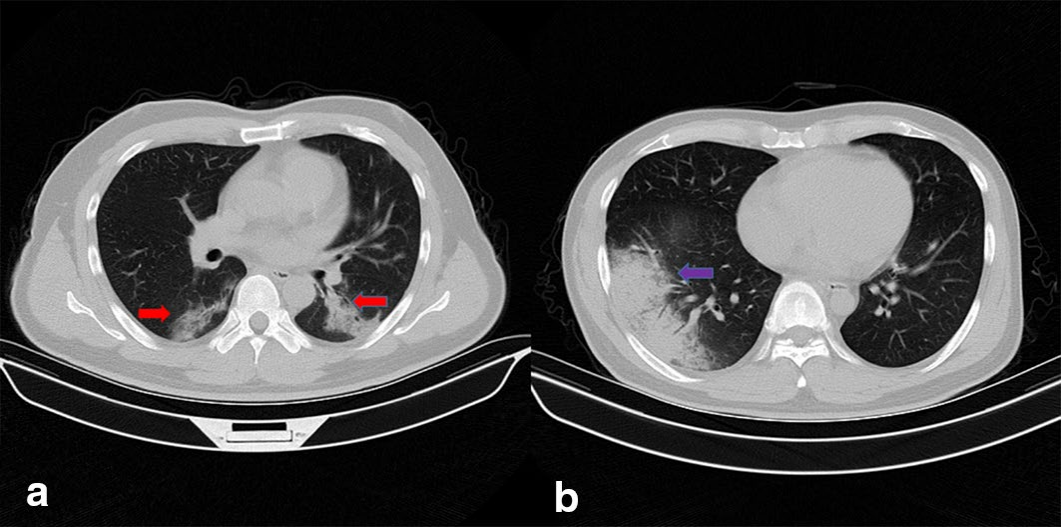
Samples of COVID-19 and GP CT images. Picture a is the CT image of COVID-19 with bilateral GGOs while picture b is the CT image of GP with unilateral GGO. The red arrows point at the GGOs of COVID-19 and the blue arrow points at the GGO of GP
